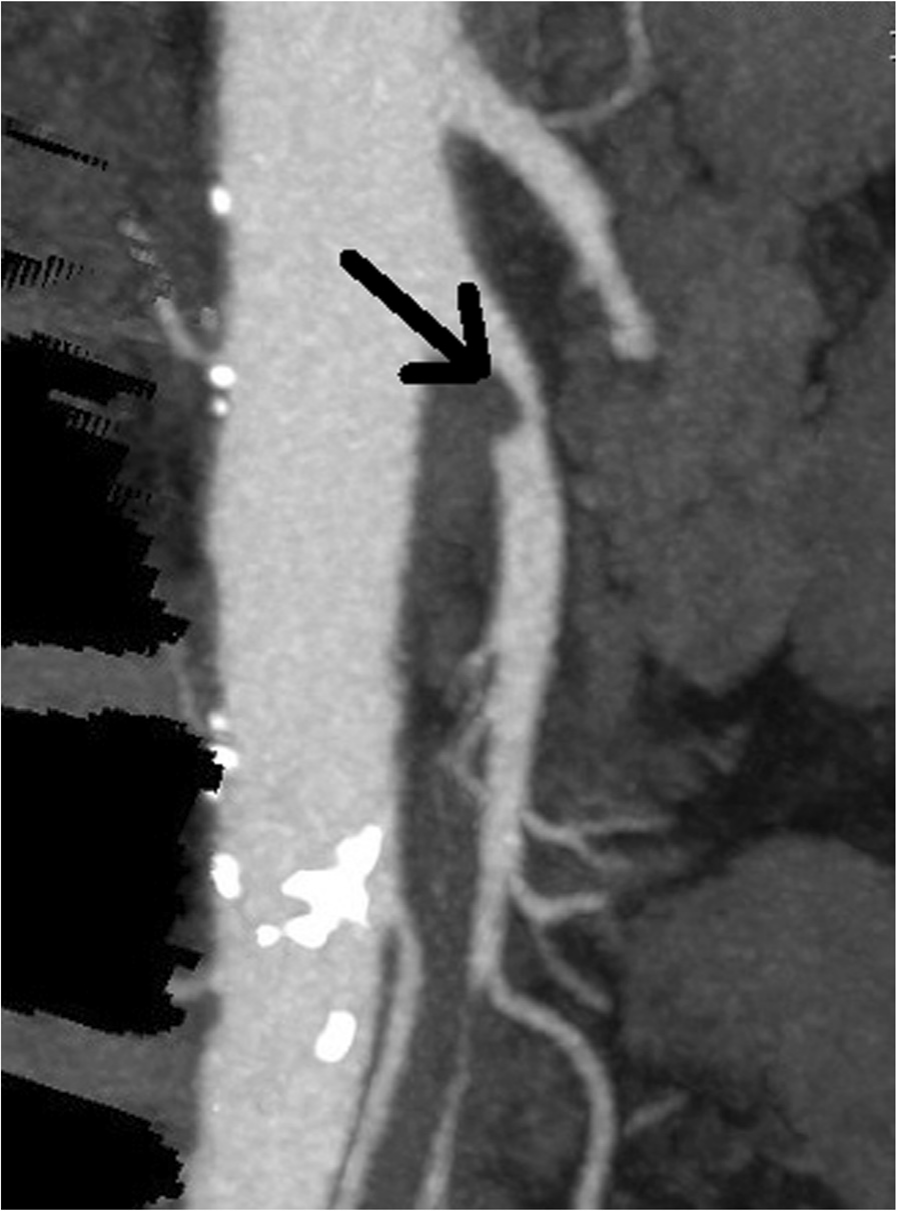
CT angiogram demonstrating stenosis of the superior mesenteric artery.
